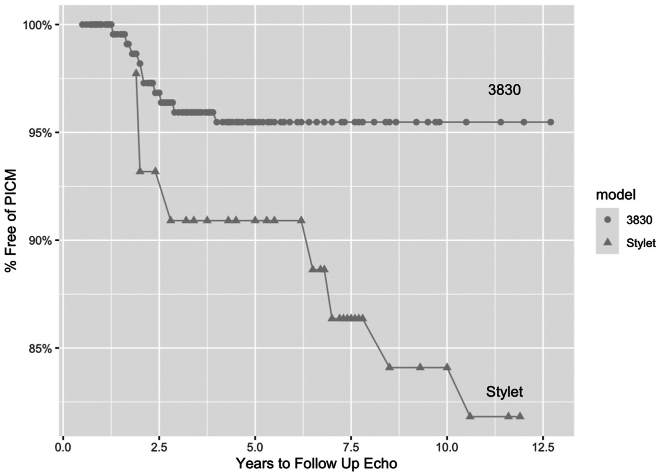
Survival free from PICM, tracked by time to diagnosis, that is, years to follow-up echocardiogram, 3830 lead cohort vs stylet-driven lead cohort (*P* = .00587). Echo = echocardiogram; PICM = pacing-induced cardiomyopathy.
